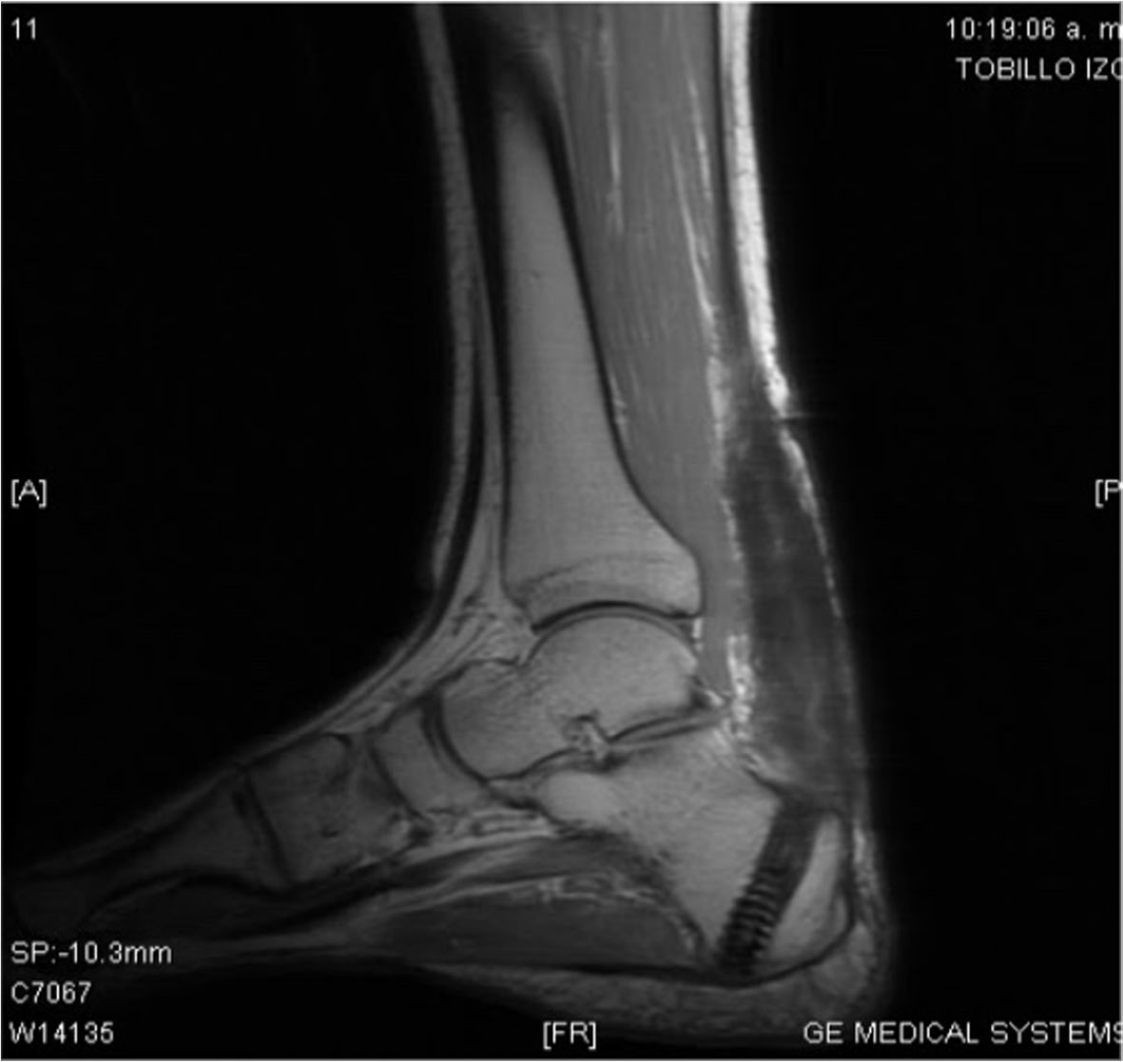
Sagittal plane MRI of left ankle - hypointense achilles tendon with fusiform appearance, enhanced by Karger's fat, hyperintense on T1 and T2 with fat saturation
